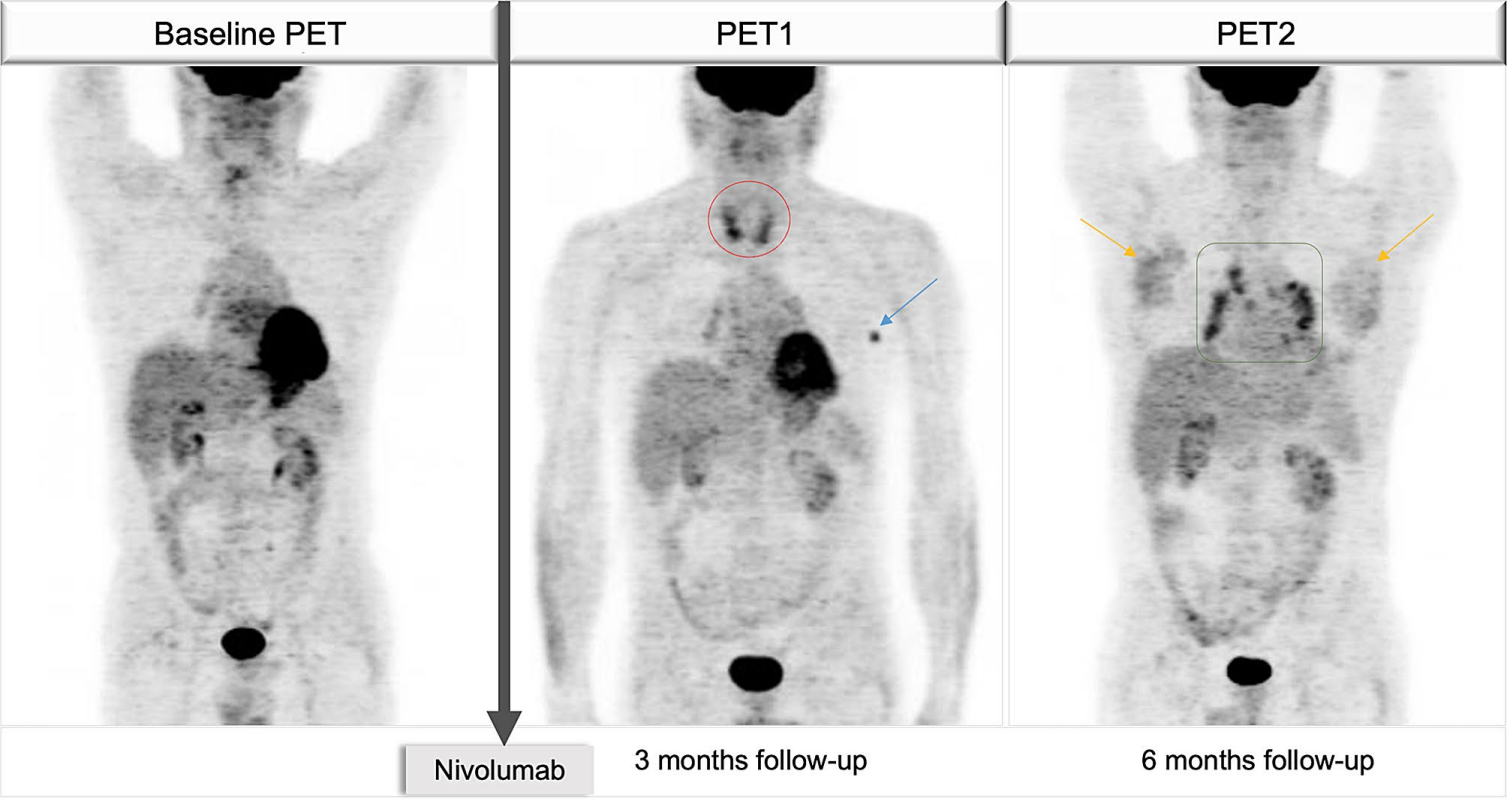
Sequential FDG PET imaging of patient #13, with stage IIIC melanoma under adjuvant Nivolumab following complete surgical resection of the primary tumor. At 3-month PET/CT follow-up an increased thyroid uptake was detected (red circle). Laboratory tests confirmed clinical hyperthyroidism, grade 2. On the same PET scan, left axillary lymph node uptake was shown (blue arrow). A biopsy confirmed the inflammatory nature of the finding. The 6-month PET scan showed a sarcoid-like reaction (green square) and increased uptake by the pectoral muscles bilaterally (yellow arrows), consistent with immune-related myositis, clinically unconfirmed
